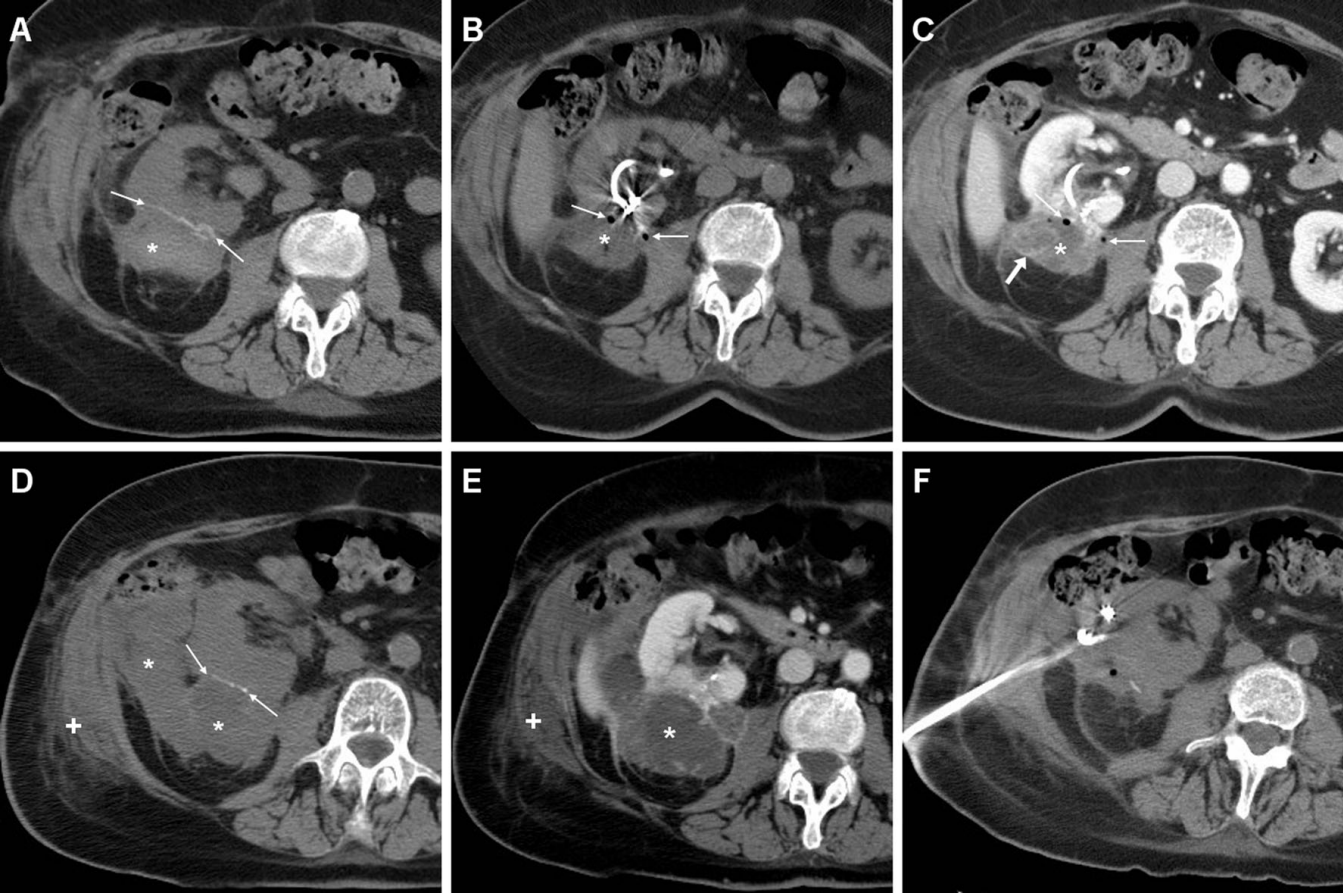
A 69-year-old woman suffering from persistent pain and haematuria 10 days after right renal tumour resection had evidence of confined peri-renal haematoma (*) abutting the site of resection indicated by sutures (*thin arrows*) on unenhanced (a) CT. After conservative treatment repeated CT (b, c—note positioning of ureteral stent) the collection (*) showed decreased attenuation, appearance of non-dependent gas bubbles (*thin arrows*) and rim-like enhancement (*arrow* in c) suggesting infection. Abscessualisation (*) was confirmed by significant size increase despite antibiotic treatment at follow-up CT (d, e) with persistent peripheral enhancement, by appearance of oedematous inflammatory thickening of the ipsilateral abdominal wall (+), and was relieved by percutaneous drainage (f)
